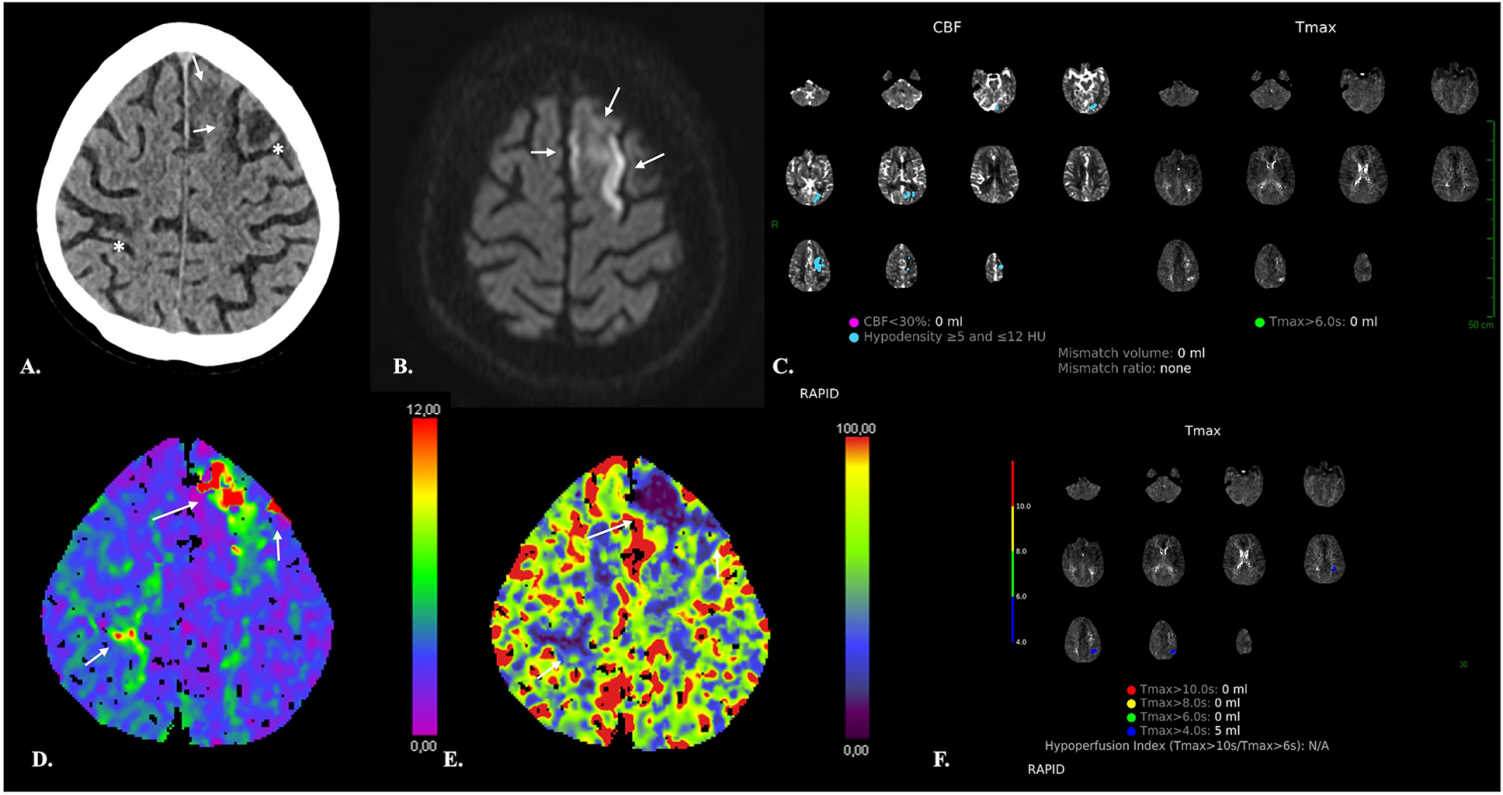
Confusing CTP features of acute superimposed on late-stage ischemic changes in an 89-year-old male with abrupt onset of left upper and lower limb paresis. (A) NECT of the brain demonstrates right rolandic and left middle frontal cortical hypodensities consistent with late-stage ischemic changes (*) and ill-defined, moderate hypodensity of the cortex and subcortical white matter of left superior frontal gyrus (*arrows*). (B) MRI performed 4 days after the CT scan demonstrates restricted diffusion consistent with acute ischemia (*arrows*). (C,F) The RAPID software discloses no corresponding NVT or TAR but demonstrates moderate left parietal hypoperfusion which is conversely MRI-negative. (D,E) Qualitative assessment of the SyngoVia CTP maps reveals changes in the Tmax and CBF in both recent and chronic lesions (*arrows*), which may be therefore difficult to distinguish on the basis of CTP only.
